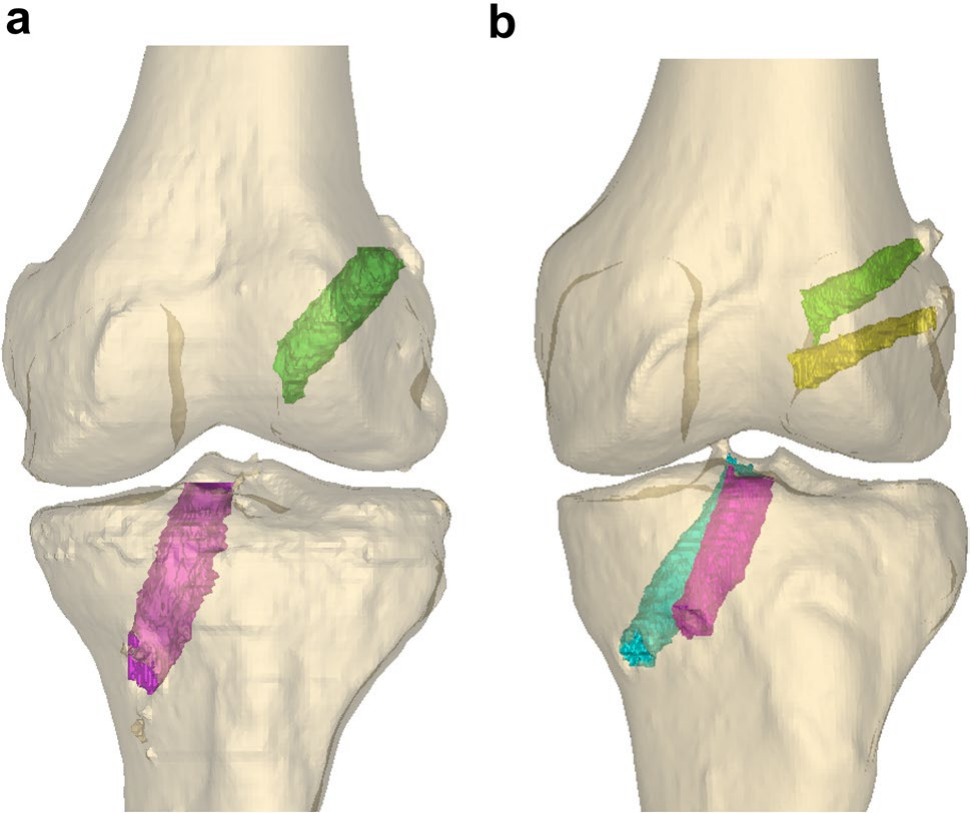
a Single-bundle ACL reconstructed knee in a 3D CT model with one tunnel on each side of the joint. b Double-bundle ACL reconstructed knee in a 3D CT model with 2 tunnels in the femur AM (*green*) and femur PL (*yellow*) and two tunnels in the tibia AM (*fuchsia*) and tibia PL (*cyan*)
